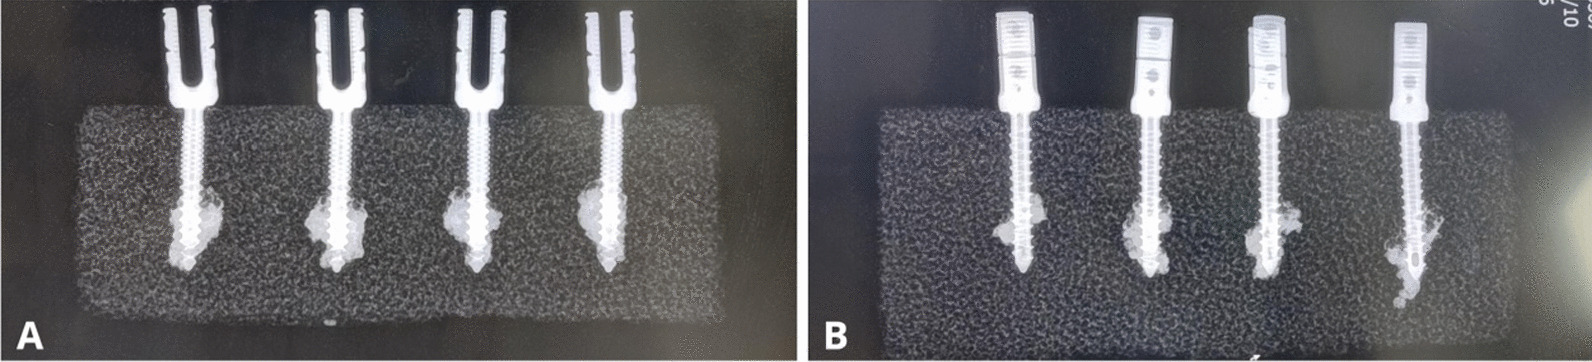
CT scans of PMMA distribution around the second-generation (A) and first-generation (B) cement-injectable cannulated pedicle screws
